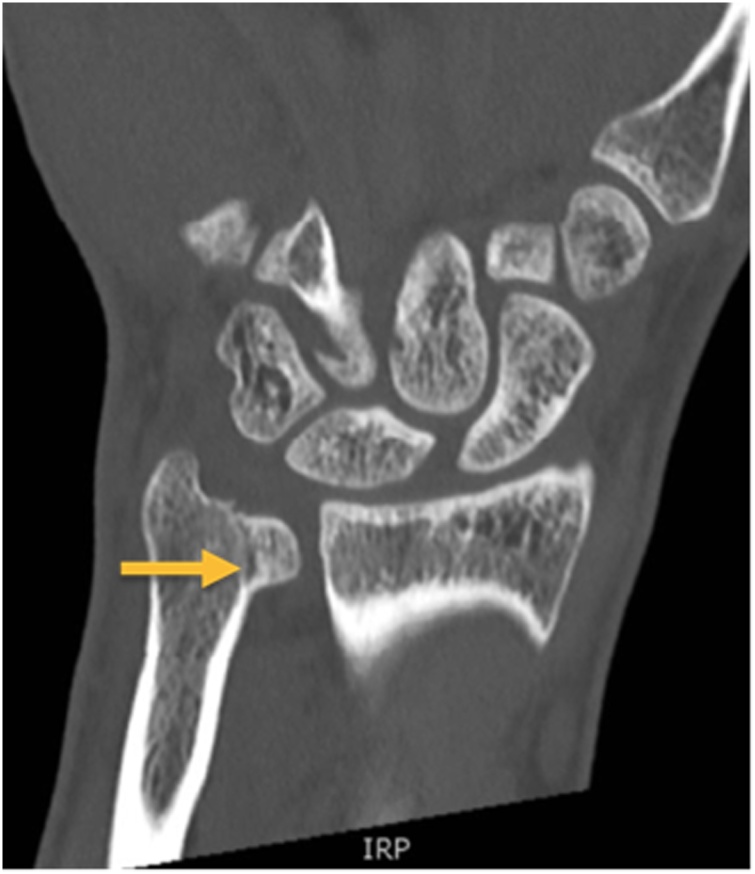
CT scan of the patient`s left wrist (coronal view) showing an undisplaced Fracture of the ulna styloid (arrow).
